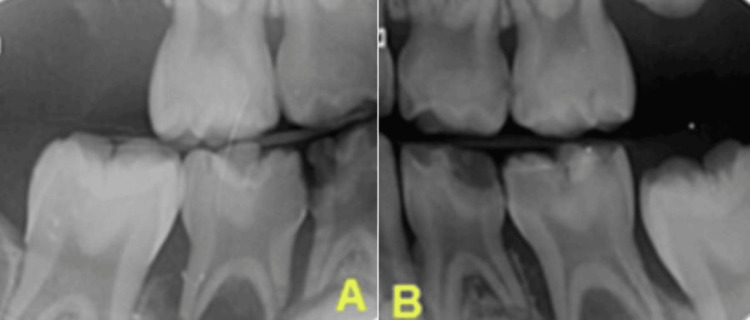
Pre-operative (a) left and (b) right molar bitewing radiographs
